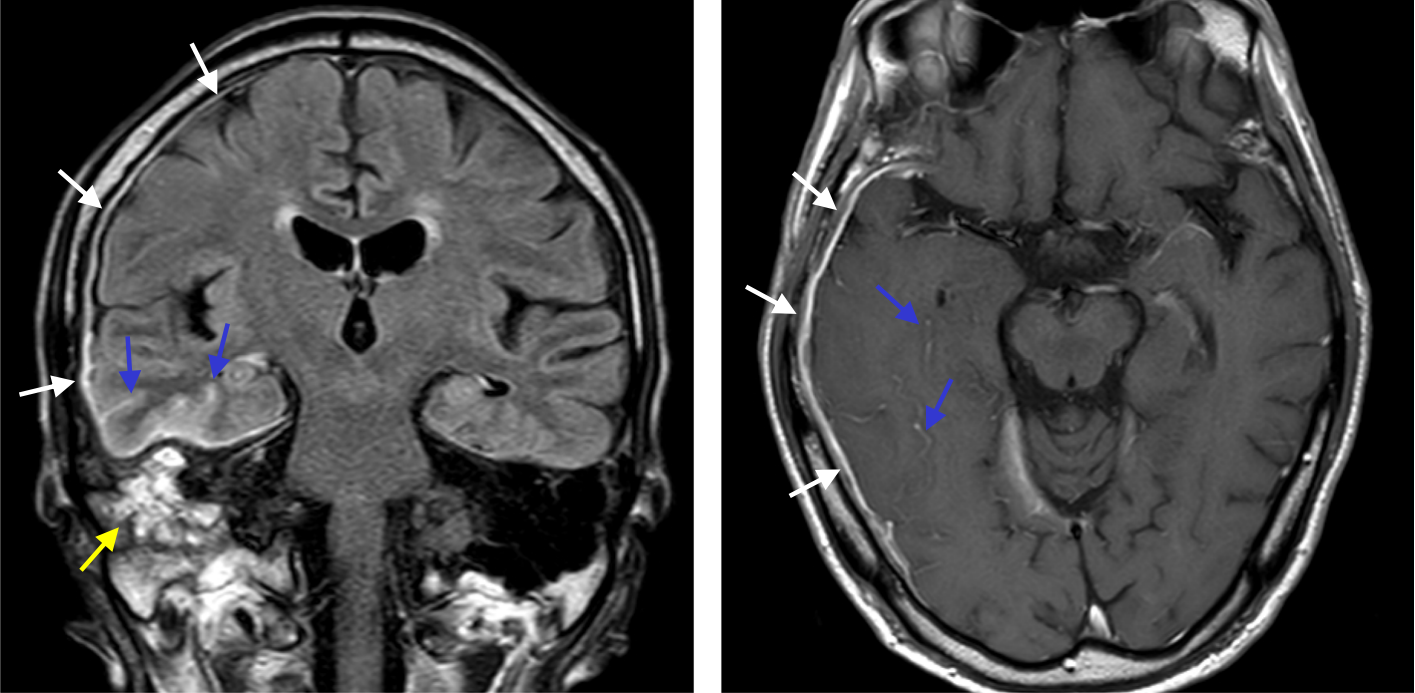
Hypertrophic pachymeningitis. Coronal section (left) of post-contrast fluid-attenuated inversion recovery (FLAIR) on MRI, and axial section (right) of post-contrast T1 on MRI. Gadolinium enhancement of the dura mater in the right temporal lobe and the middle cranial fossa (white arrows), and enhancement of the pia mater (blue arrows) Right mastoiditis was also evident (yellow arrows)
